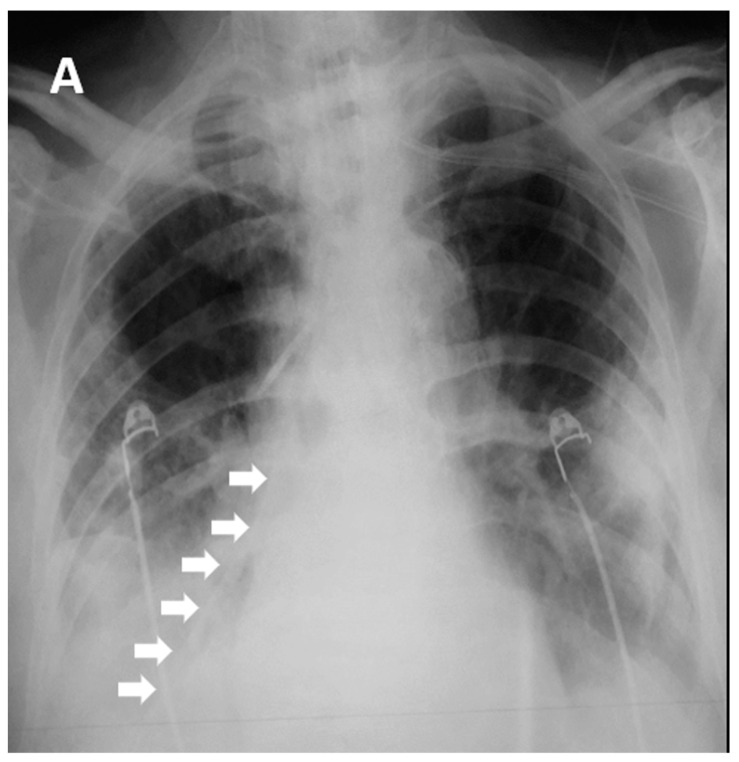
Chest X-ray in one of the first COVID-19 ARDS patients admitted in our ICU. The patient was turned from prone to the supine position on the 4th ICU day, late in the night, and nasogastric tube position was checked with palpation of a “flash” of air in the epigastrium, although there could not be observed any aspirated fluid. Enteral nutrition was started. Eight hours later the patient became hypoxemic, and increased tracheobronchial secretions were noted. A chest X-ray was ordered which revealed the NGT mispositioning in the right lower lobe. White arrows indicate the misplaced nasogastric tube in the Right Lower Lobe.
